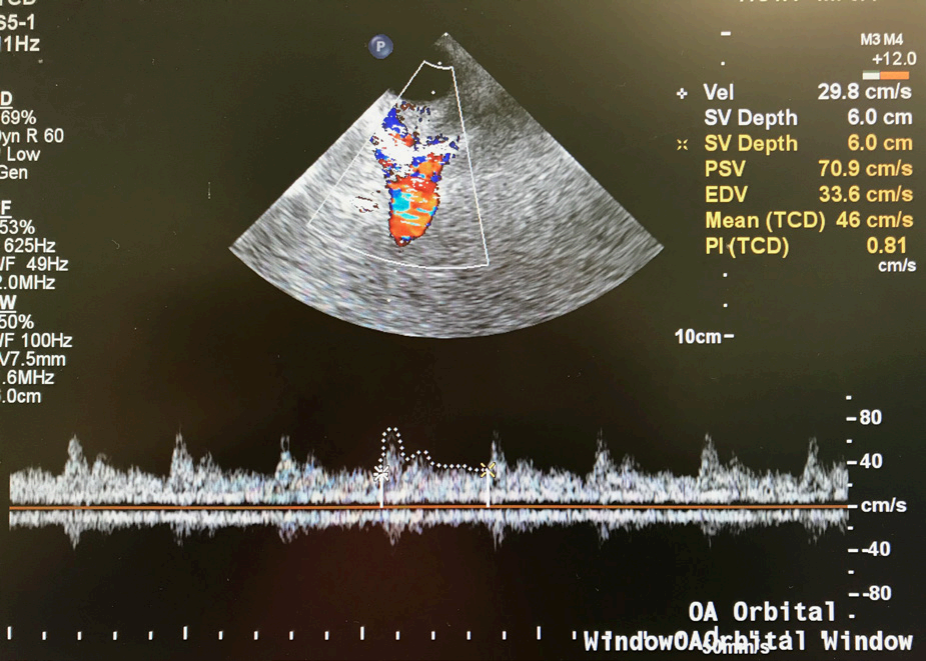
Colour Doppler imaging of the ophthalmic artery, at the end of the operation. OA mean velocity: 46 cm sec^−1^.
